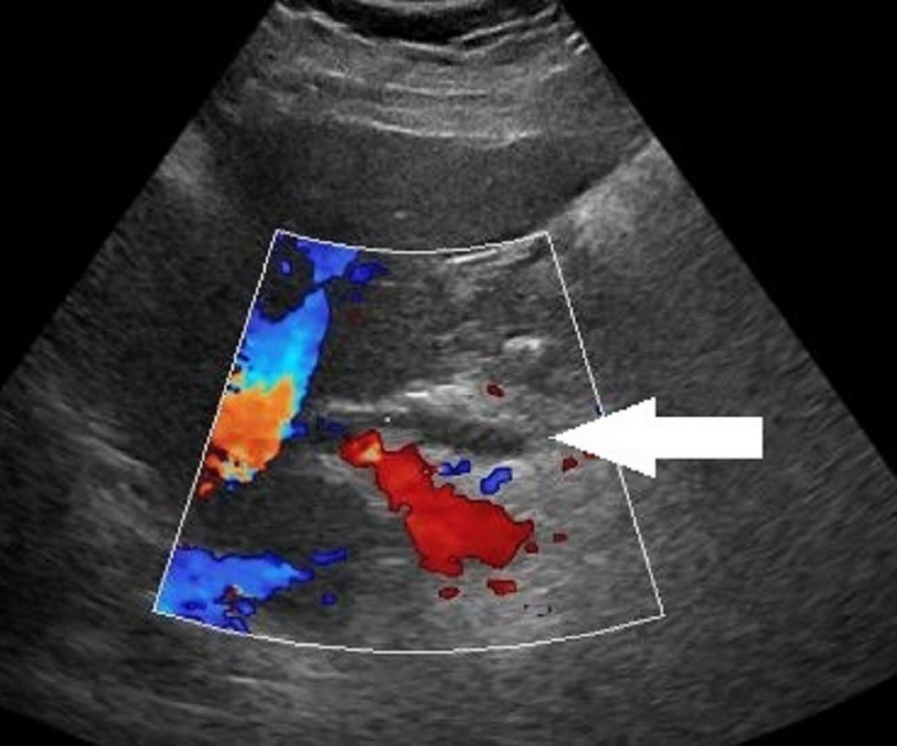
Hepatic ultrasound visualized mild heterogeneous increase (white arrow) in echogenicity of the liver, consistent with mild fibrotic change
